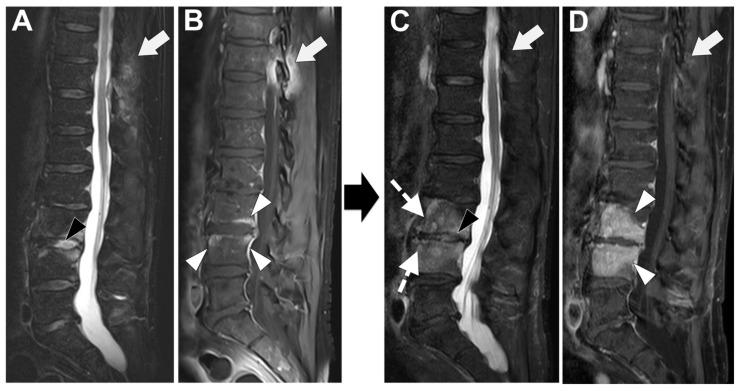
A 73-year-old male presented with a rapidly evolving lumbar pain and fever, with an inflammatory syndrome on blood samples. A first MRI was performed as infectious spondylodiscitis was suspected, which included (A) STIR T2-weighted imaging (WI) and (B) fat sat T1-WI after gadolinium chelates injection. It shows a high signal intensity (SI) of the L3-L4 disc while other disks are in lower signal (black arrowhead), as well as linear subchondral contrast enhancement (CE) of the L3-L4 endplates both linear and more pronounced at the upper anterior corner of the L4 vertebral body (white arrowheads). Moreover, the left T11-T12 facet joints displayed marked edema of the subchondral bone and surrounding tissues (white arrows). A control MRI with SITR T2-WI (C) and fat-suppressed CE-T1-WI (D) was performed one month later, demonstrating a marked narrowing of the L3-L4 disk (black arrowhead), erosions of the vertebral body (dashed white arrows), extensive edema in the L3 and L4 vertebral body (white arrowhead), a persisting arthritis involving the left T1-T12 facet joint. *Bacillus cereus* was found on the Bacterial analysis of the L3-L4 disk biopsy.
